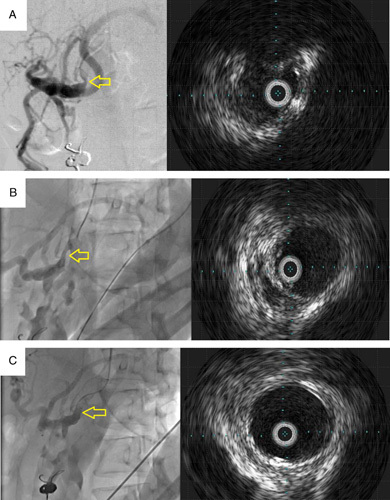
Interventional radiology and intravascular ultrasonography before and after incision of the median arcuate ligament. Interventional radiology and intravascular ultrasonography after coil embolization of pancreatoduodenal artery aneurysm (A). After coil embolization, the celiac artery was still compressed by the median arcuate ligament, and intravascular ultrasonography showed evidence of stenosis. After median arcuate ligament incision, interventional radiology and intravascular ultrasonography were performed again (B). The incision resulted in the dilation of the celiac artery and lumen of the vessel. The celiac artery was additionally balloon dilated. Interventional radiology and intravascular ultrasonography were performed after dilation (C). We determined that the hemodynamic abnormalities associated with median arcuate ligament had improved after confirming adequate celiac artery dilation.
